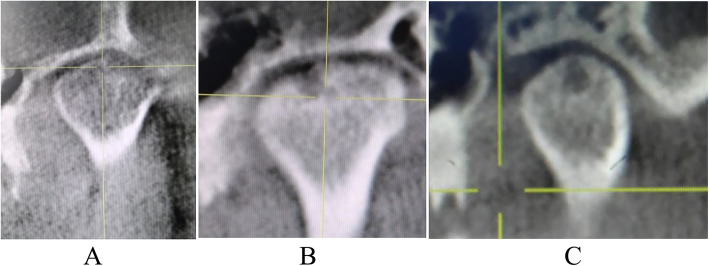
CBCT manifestations of TMJOA patients. a: TMJOA with condylar bone defect of surface; b: TMJOA with obvious condylar defect; c: Cystic degeneration in coronal image
